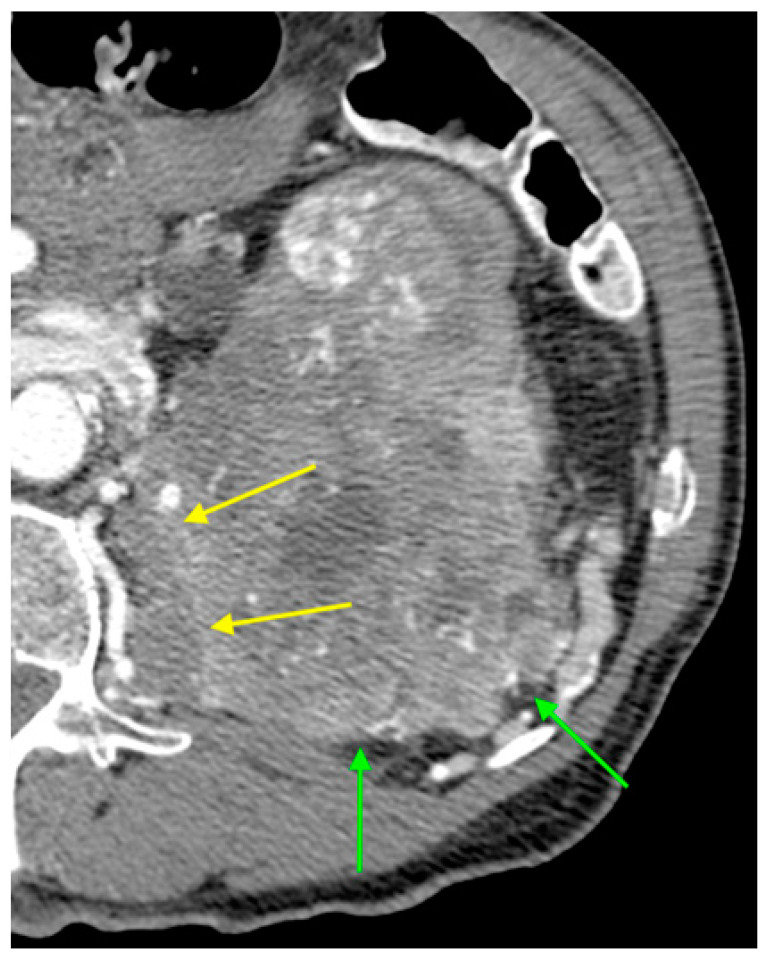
Axial CT image during arterial phase showing ccRCC with GIMAP genes expression with absence of endophytic growth pattern (green arrows) and signs of infiltration of the left psoas major muscle (yellow arrows).
